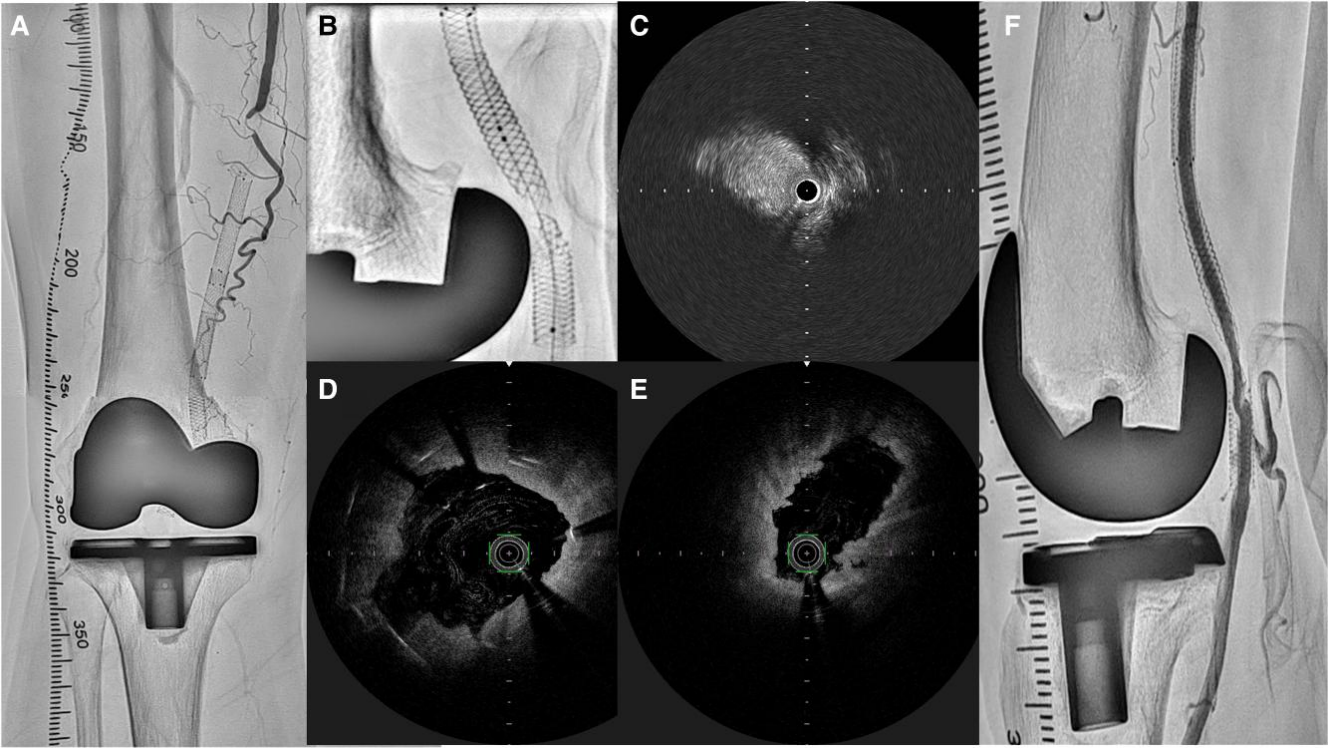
Imaging studies of the fourth endovascular treatment. (*A*) Angiography showed occlusion from the superficial artery to the popliteal artery. (*B*) Fluoroscopy showed Supera stent fracture at the site closest to the artificial knee joint. (*C*) Optical frequency domain imaging demonstrated Supera stent fracture with partial protrusion into the lumen and (*D*) areas not covered by the stent. (*E*) Intravascular ultrasound showed a lesion with low stent coverage and vessel dilation. (*F*) Final angiography confirmed blood flow, but vessel dilation was inadequate.
